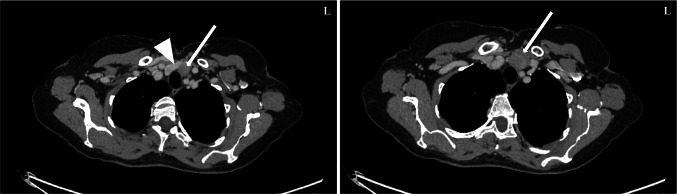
Preoperative computerized tomography (CT) scan of the superior thoracic inlet. The thyroid gland is marked by a white arrowhead, and the white arrow designates the adjacent 24 mm lesion originally believed to constitute an enlarged perithyroidal lymph node in level VI. The image on the right is taken caudal to that on the left and highlights the maximum diameter of the mass, reaching the superior thoracic aperture
